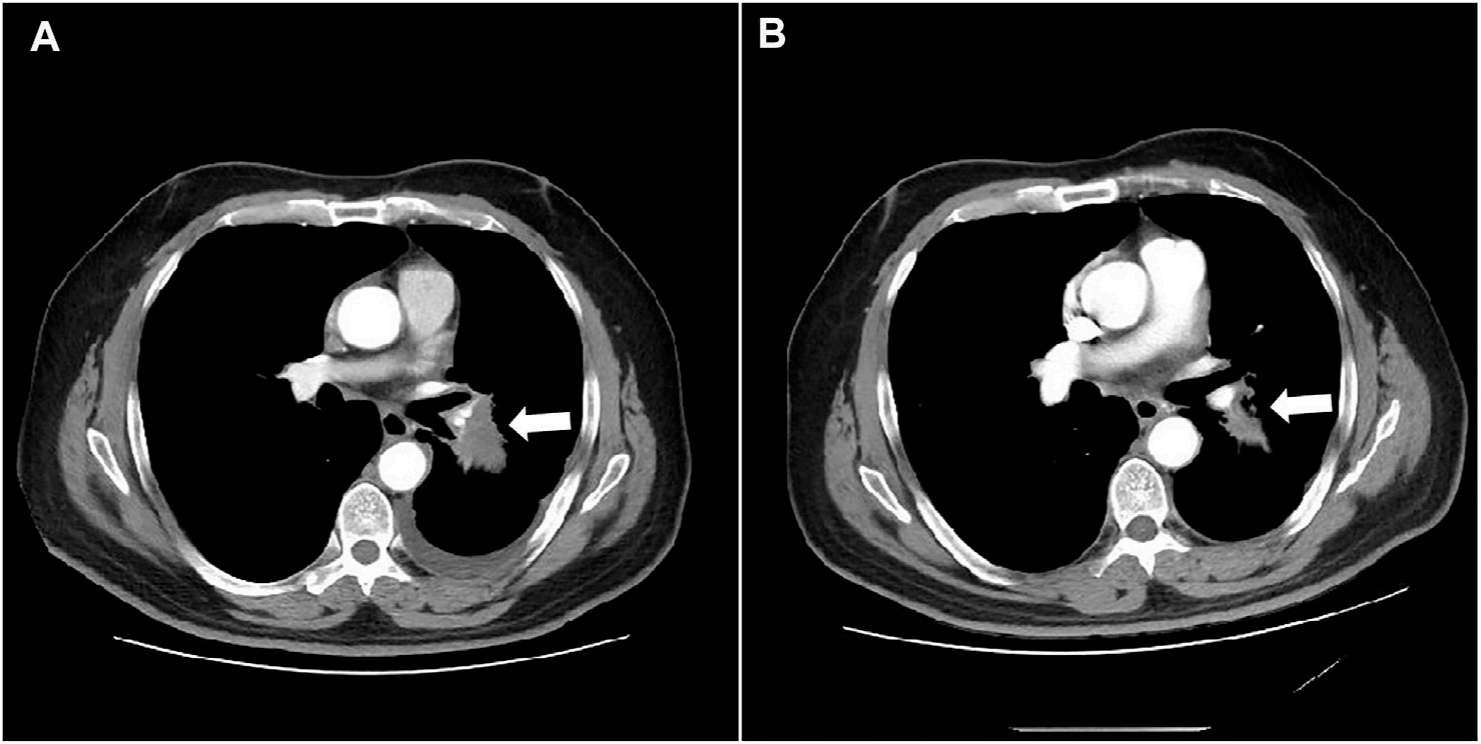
Computed tomography shows the mass in the left lung on mediastinal window before and after treatment (A) before anlotinib treatment; (B) 4 cycles of anlotinib treatment).
